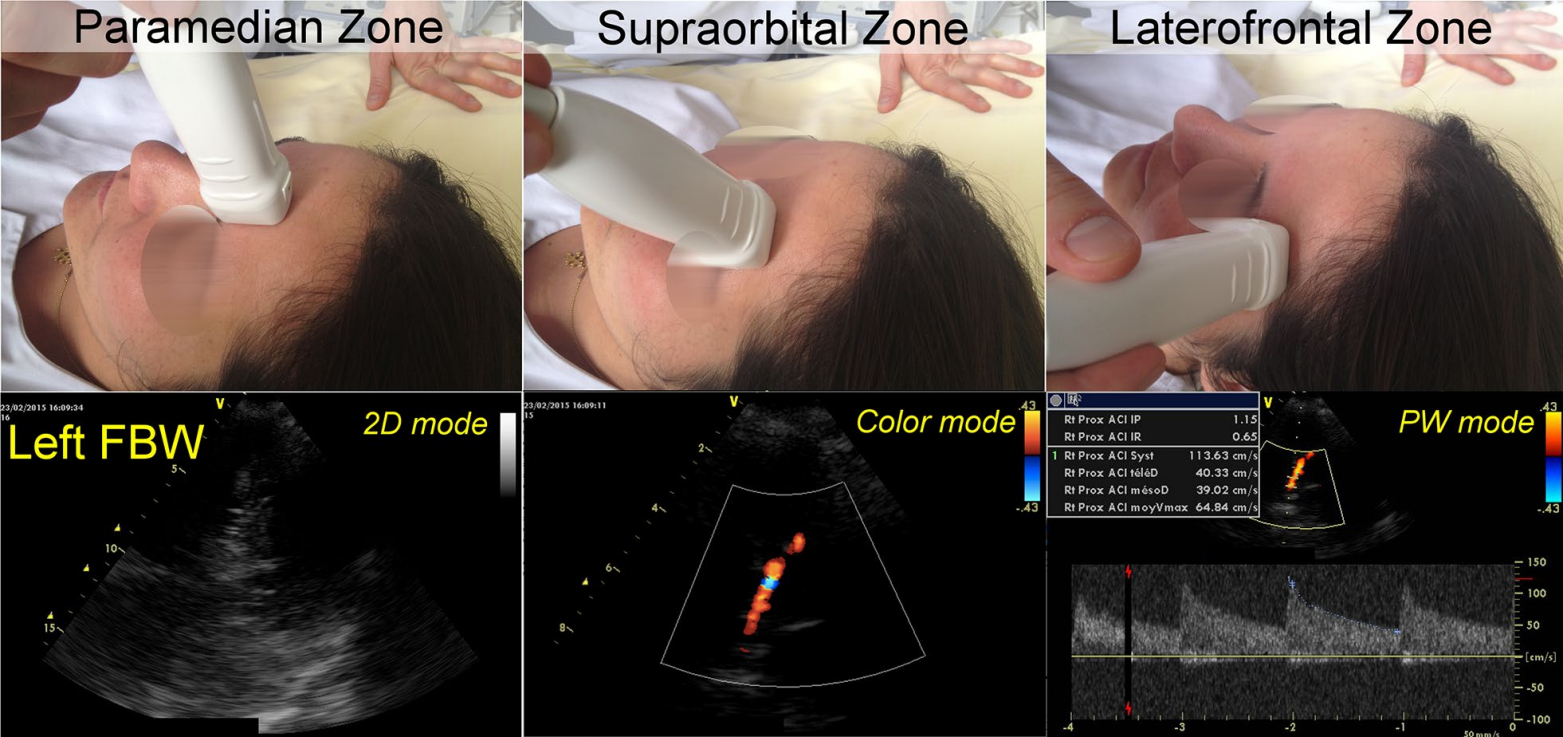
Description of the FBW technique for TCD ultrasonography. The transducer is positioned vertically at the paramedian frontal zone (index mark at the top), rotated 90° outward and shifted horizontally (index mark laterally) to the supraorbital zone at the top of the orbital arcade, up to the laterofrontal zone. Visualization of the brain parenchyma in B-mode (two-dimensional mode), insonation of the A2 segment of the ACA in color mode, and blood flow velocity measurements using the pulsed Doppler (PW) mode. [*ACA* anterior cerebral artery, *PW* pulsed waved Doppler]
